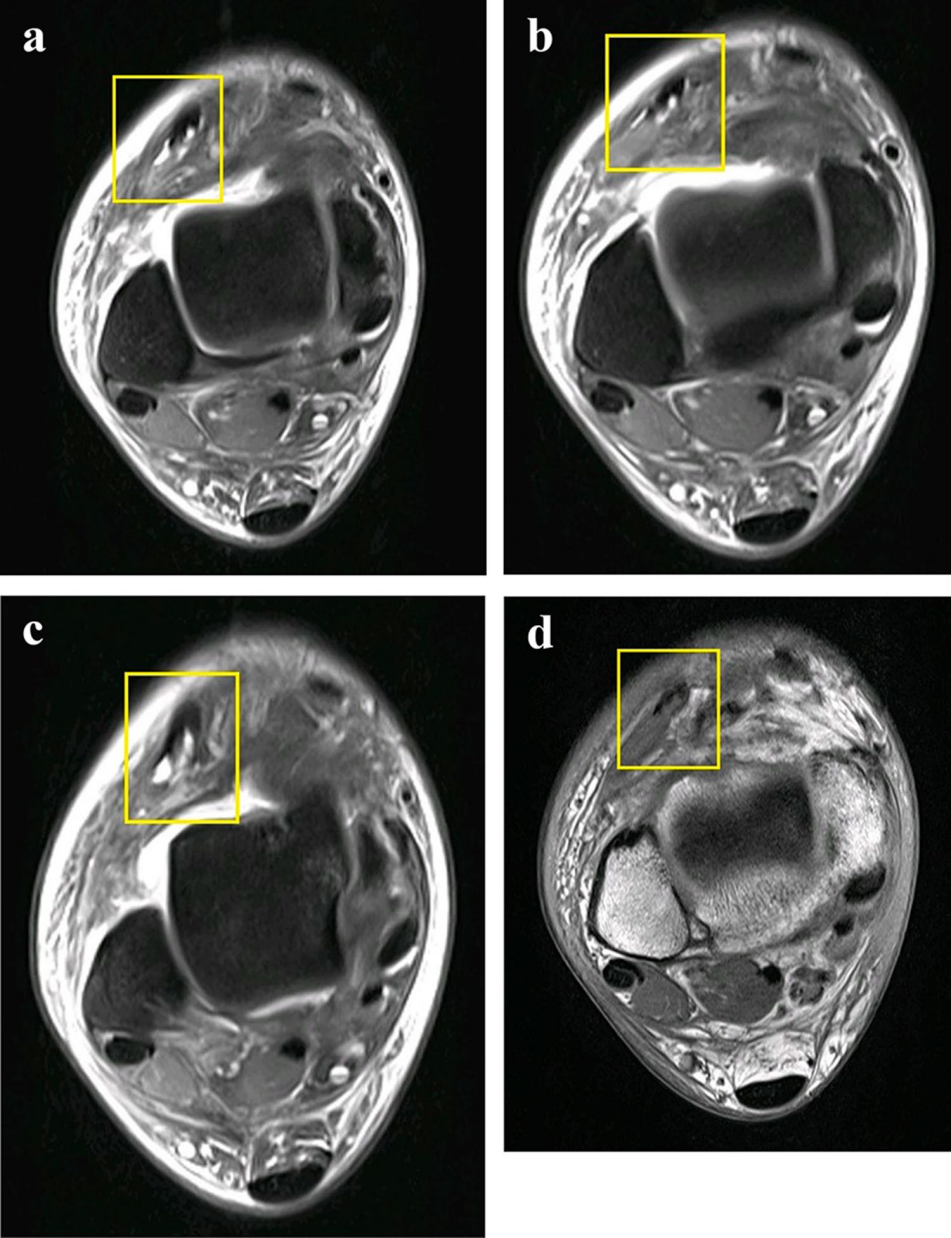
MRI T1W (a–c) and PDFS (d) axial images showing heterogenicity of the Extensor Digitorum longus (yellow box) and partial tear near the Myo-tendinous junction. The epimysium is inconspicuous. The hernia is not seen
